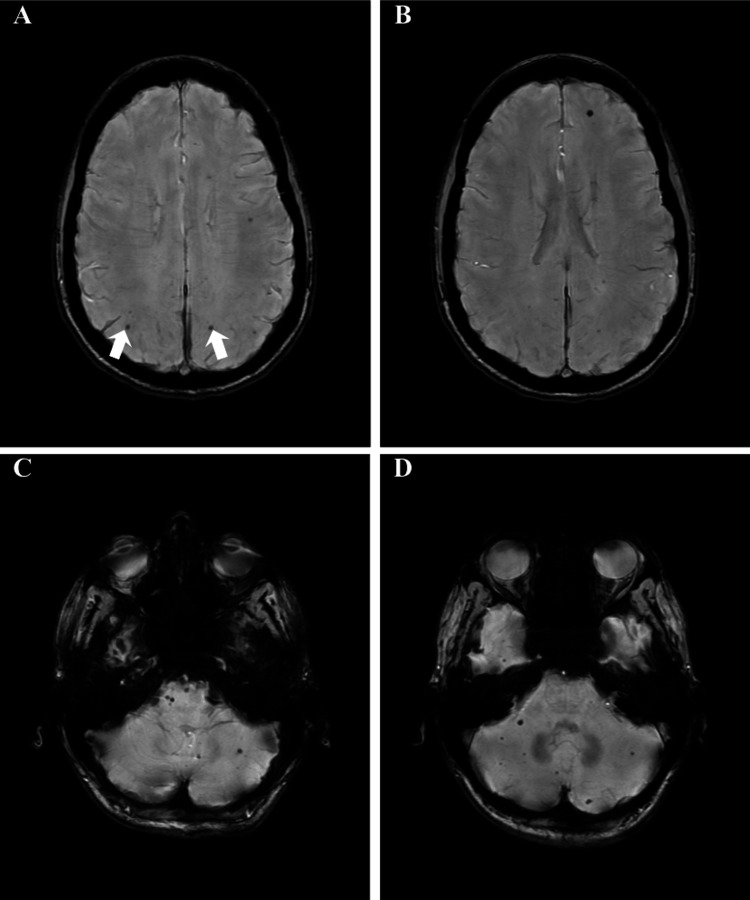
Susceptibility weighted images on MRI of the brain. A: One cavernous malformation is seen in each occipital-parietal lobe, indicated by the white arrows. B: A cavernous malformation is seen in the left frontal lobe. C and D: There are several cavernous malformations seen throughout the brainstem and cerebellum. While the typical appearance of cavernous malformations is mulberry-like clusters, the lesions in this patient are more visually consistent with several microhemorrhages, classifying them as microcavernomas. The radiology report for these MRI slices in this figure included both microhemorrhage and cavernous malformation on the differential diagnoses list. Given this, genetic testing was ordered to assess for familial cerebral cavernous malformation.
